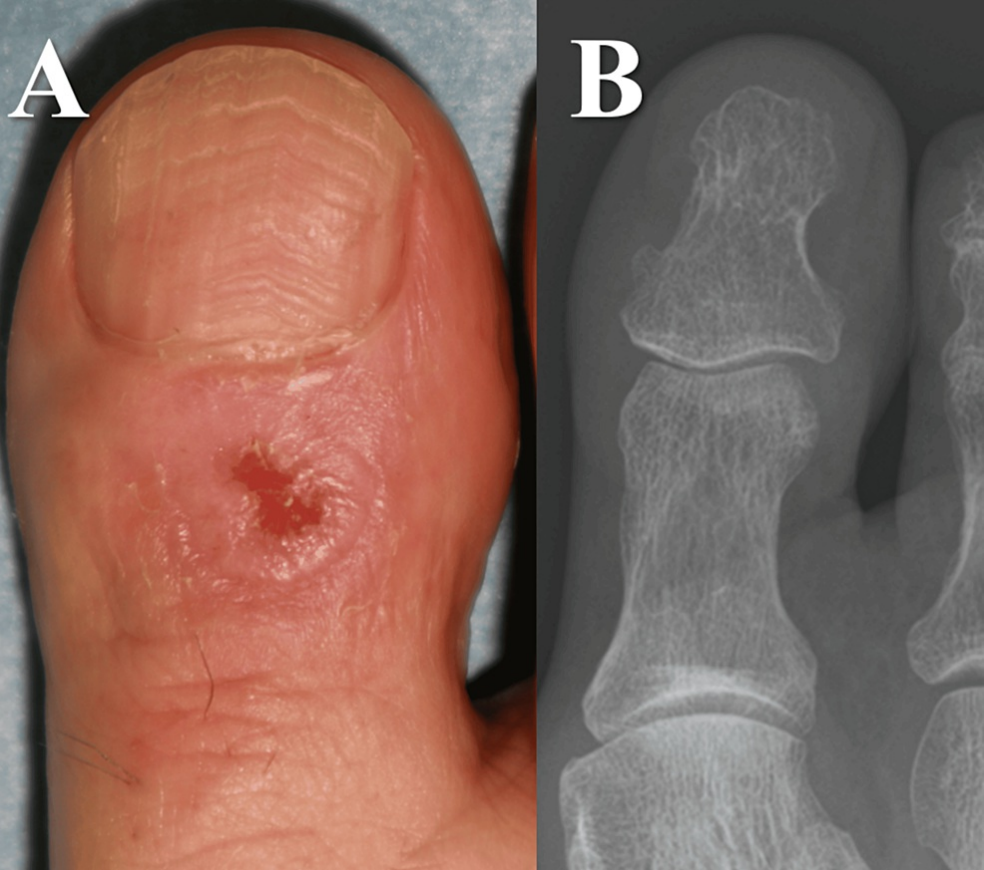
Preoperative clinical photograph and radiographic findings (Case 2) (A) A cyst was identified on the dorsal side of the IP joint. It appeared dry and shriveled. (B) Anteroposterior radiographs of the right hallux show no remarkable changes.
